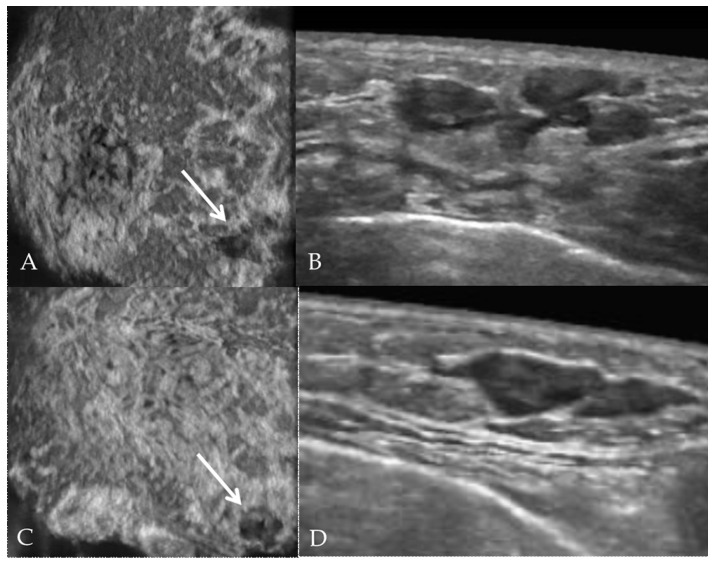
Automated breast ultrasound scan on coronal (A,C) and axial planes (B,D). The lesion appears as an oval hypoechoic nodule with regular edges, mimicking duct ectasia (arrows).
